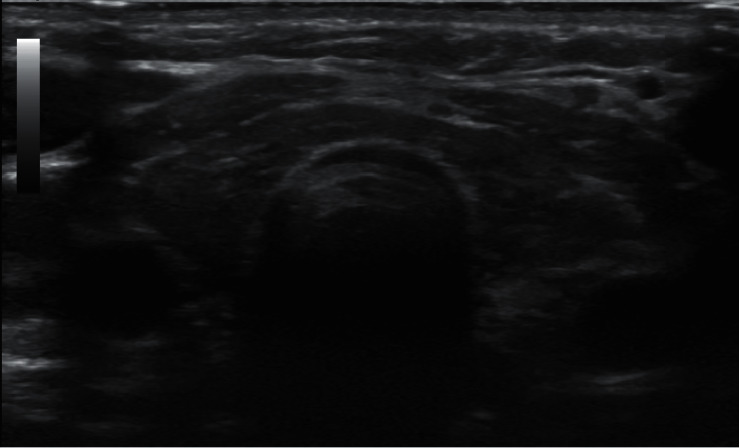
Thyroid ultrasound showing the relatively small size of the lobes and isthmus, diffuse coarse, heterogenous parenchymal echotexture with multiple fibrous echogenic lines, and no focal nodule.
